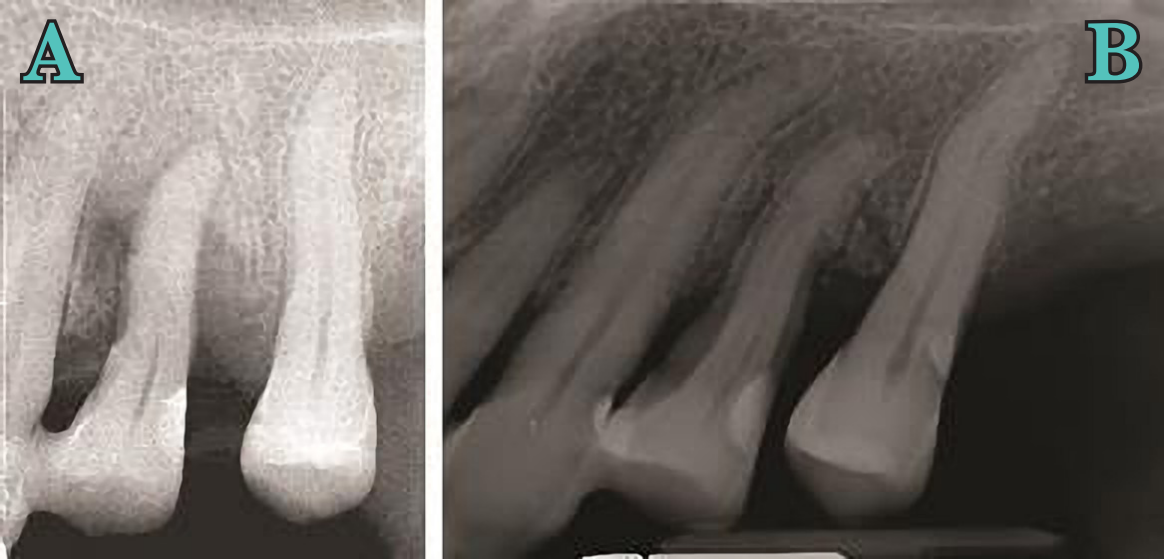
Tooth 2.4 x-ray at 3 months from the initial examination - periodontal (A) and radiographic examination (B).
